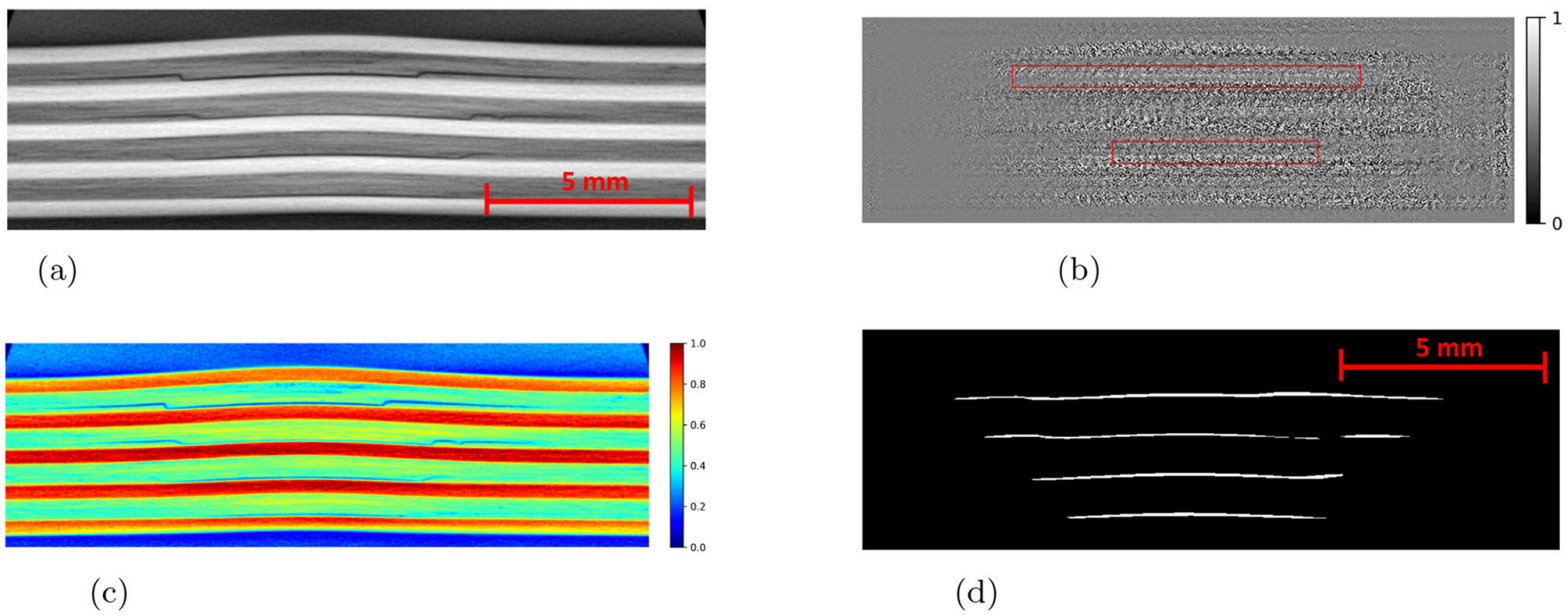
Visualization of results for impact energy of 7.5 Joules. (c) Shows the attribution map produced using the IG method applied on the trained Detectron2 model, using the CT slice of the FML plate shown in (a). The model's predicted mask is displayed in (d), highlighting its interpretability. Example red boxes shown in attribution map (b) highlight attribution of damages. (a) CT slice with impact energy 7.5 Joules. (b) Attribution map. (c) Attribution map (modified loss). (d) Predicted mask by Detectron2.
